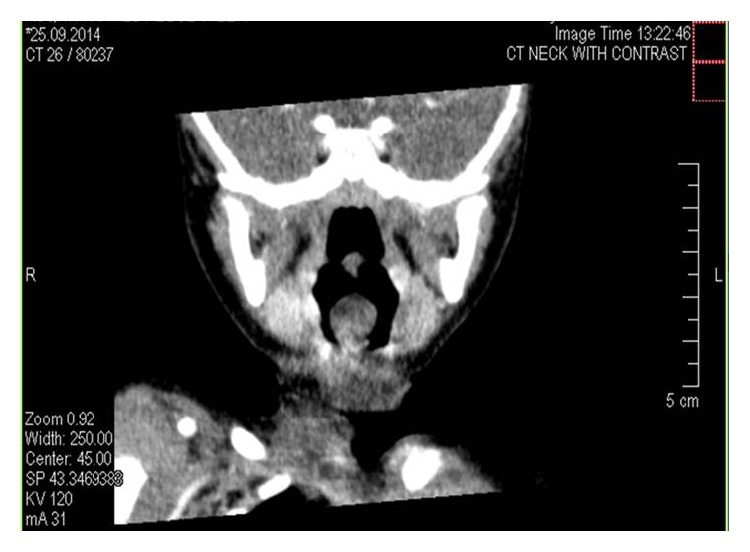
Coronal CT scan showing retroglossal fairly defined rounded nonenhancing hypodense cystic lesion measuring about 9 mm, seen projecting into oropharynx.
